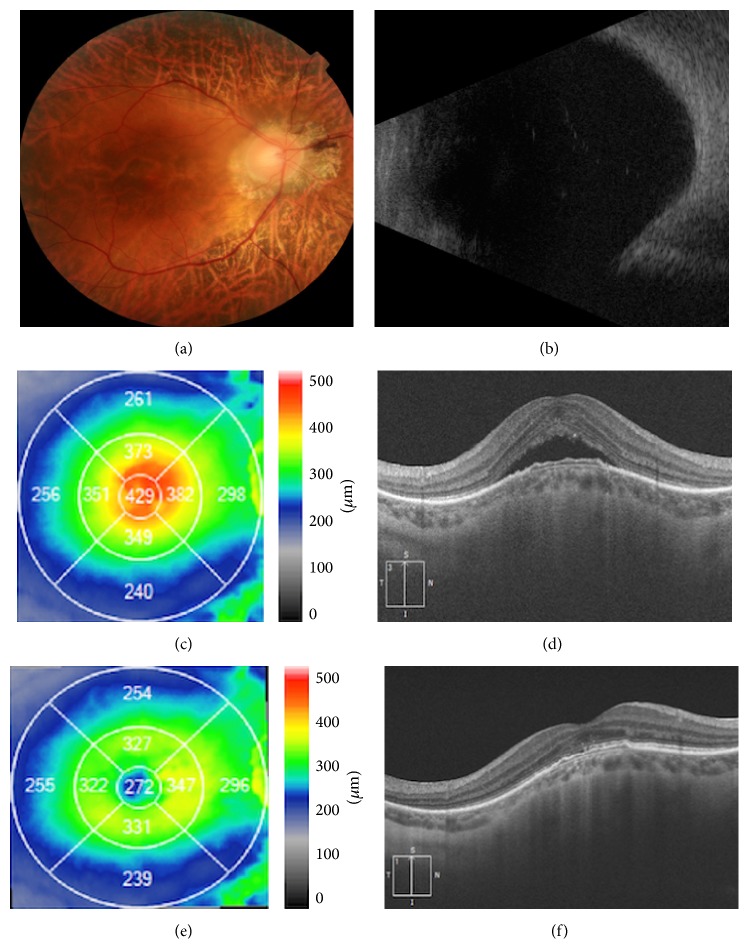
Dome-shaped macula with serous retinal detachment before and after treatment with spironolactone. (a) Color photo showing peripapillary atrophy, marked retinal thinning with visible choroidal vessel, but no hemorrhage in the foveal area. (b) B-scan ultrasound showing an abnormal ocular wall in the posterior fundus. (c) Pretreatment OCT macular map showing increased CRT. (d) Pretreatment OCT image showing serous retinal detachment in the foveal area with no other alterations. (e) Posttreatment OCT macular map showing improved CRT. (f) Posttreatment OCT image showing resolution of the serous retinal detachment in the foveal area with an abnormal ellipsoid line.
